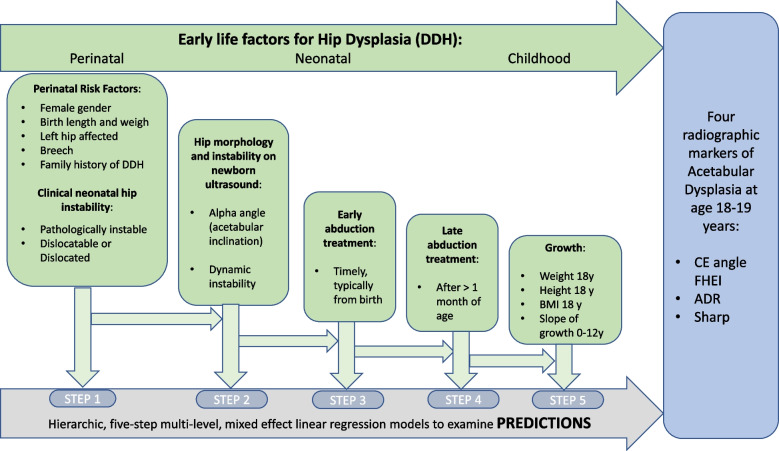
The statistical five-step hierarchic model used to analyze how early life factors can predict four radiographic measurements of acetabular dysplasia at 18–19 years of age. DDH Hip dysplasia. CE angle Center edge angle of Wiberg. FHEI Femoral head extrusion index. ADR Acetabular depth-width ratio. Y years old
